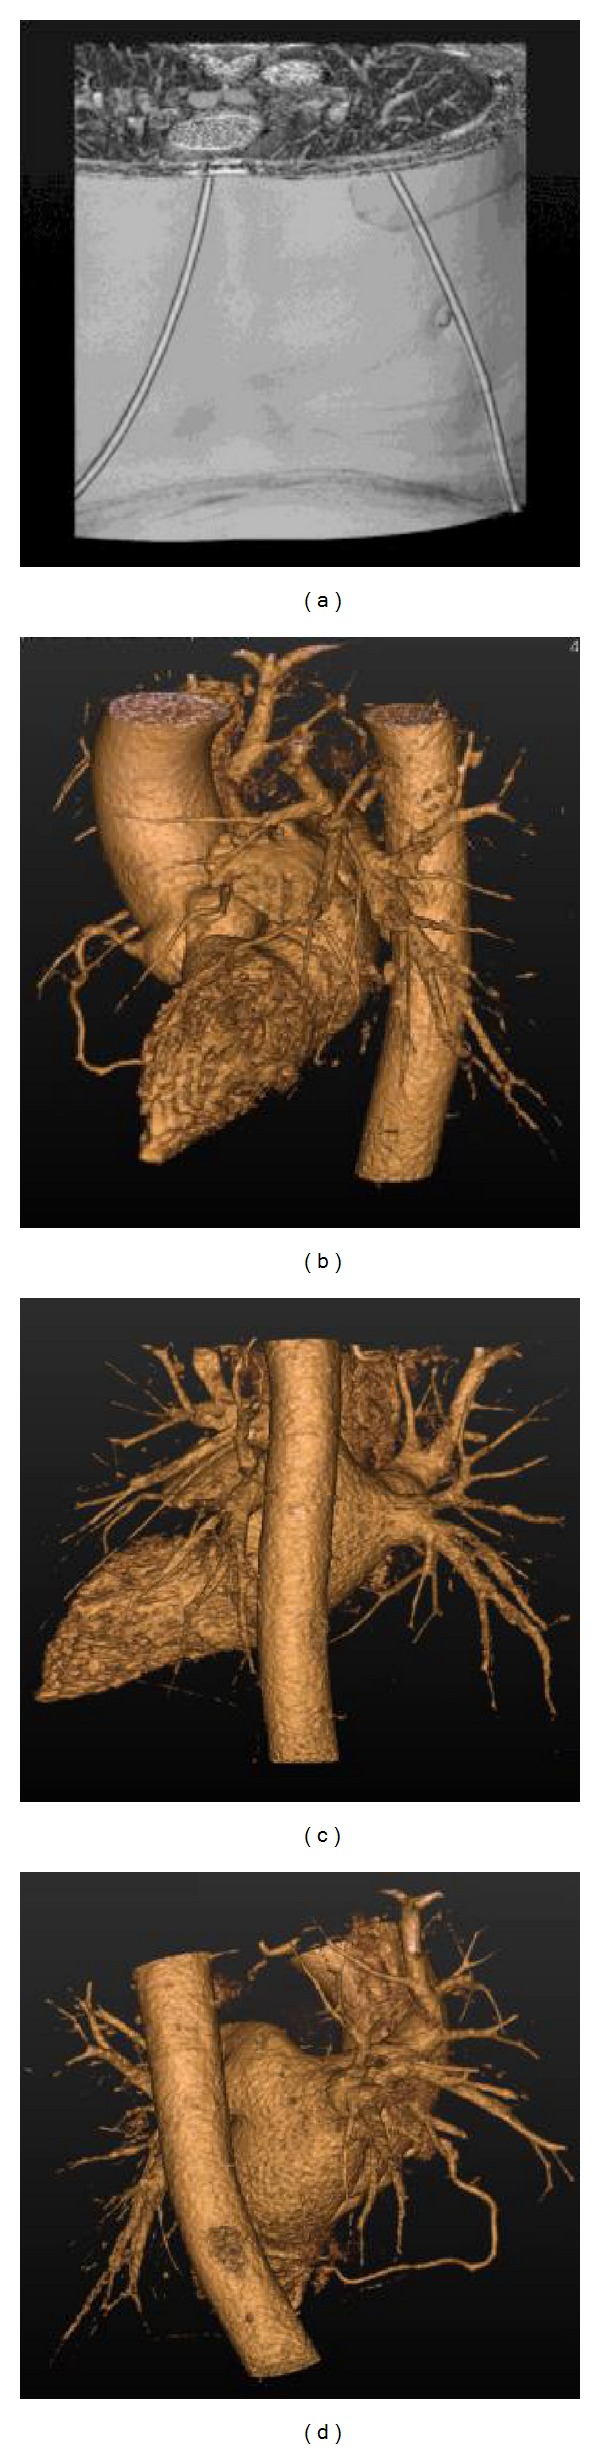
3D original dataset and the extracted vessel trees with different points of view. (a) 3D cardiac CT angiography dataset; (b), (c), and (d) cardiovascular trees from three different points of view.
